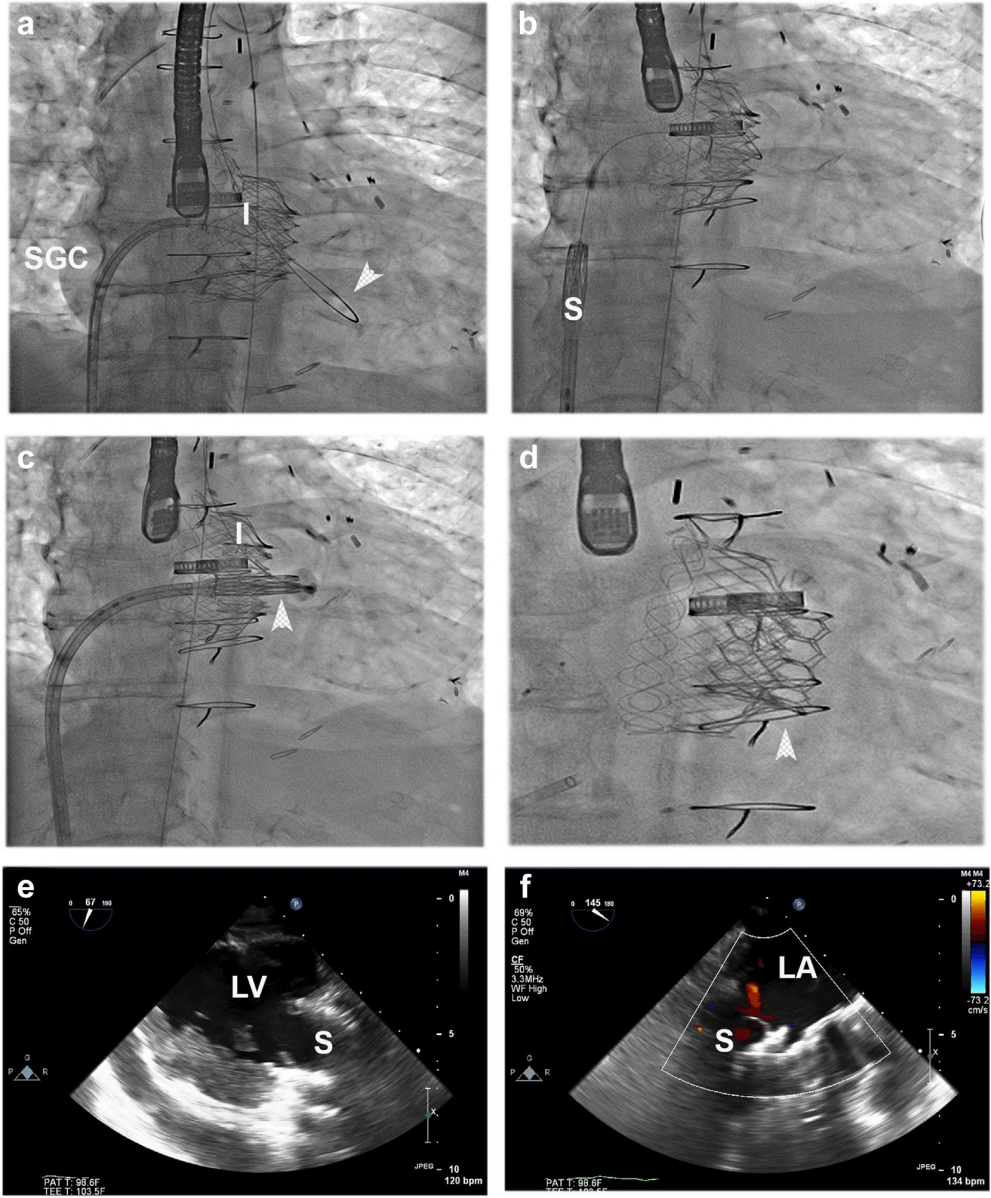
Mitral valve-in-valve therapy for acute malposition of the Intrepid prosthesis. (a) To minimize the risk of movement of the Intrepid prosthesis that could lead to inversion, a rail (arrowhead) was created using a steerable guide catheter (SGC). (b) Tension on the rail helps to ensure passage of the Sapien (S) prosthesis without affecting the Intrepid valve. (c) and (d) To treat leaflet overhang, the ventricular side of the Sapien prosthesis was positioned one-third from the Intrepid outflow portion (arrowheads). (e) and (f) Echocardiography showed coverage of the native leaflet with the Sapien prosthesis and elimination of the MR. Abbreviations: LA, left atrium; LV, left ventricle; MR, mitral regurgitation.
